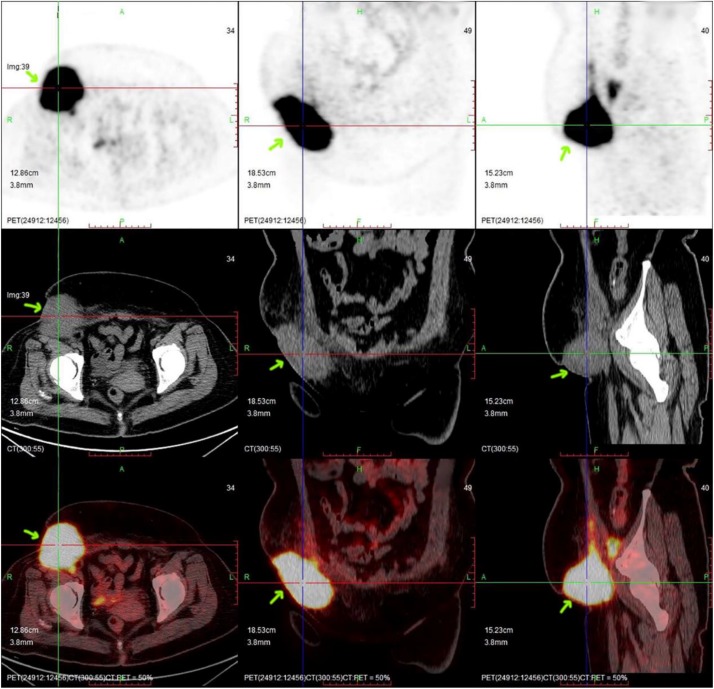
PET/CT imaging before CAR-T cell infusion. The position indicated by the arrow is the location of the lesion, which had a volume of ∼71 mm × 72 mm × 98 mm (arrow).
